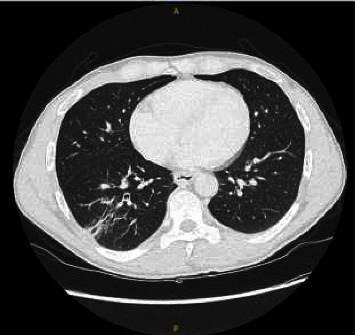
Chest computed tomography of Case 3 four months after starting treatment.
